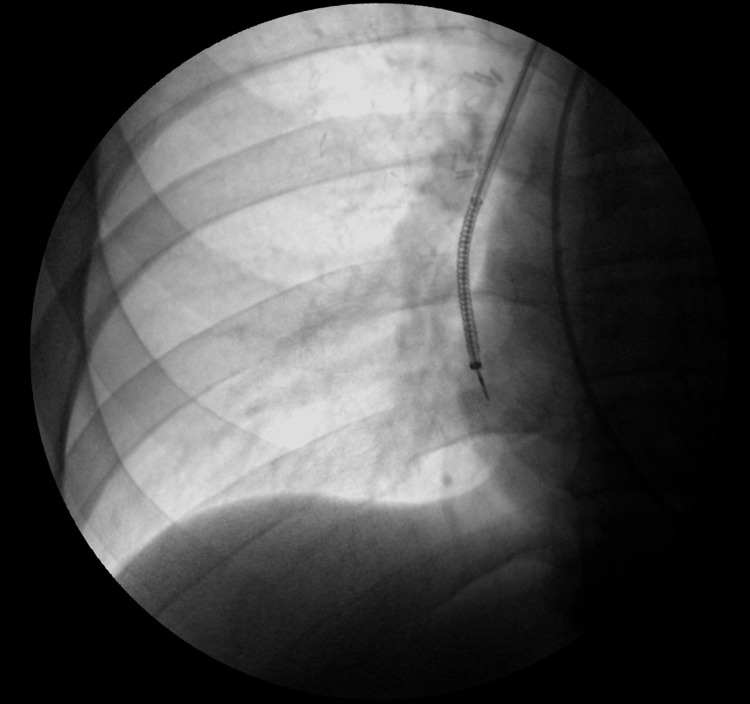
Robot-assisted bronchoscopy with right lower lobe lung nodule transbronchial needle aspiration biopsy using radial endobronchial ultrasound and fluoroscopy
